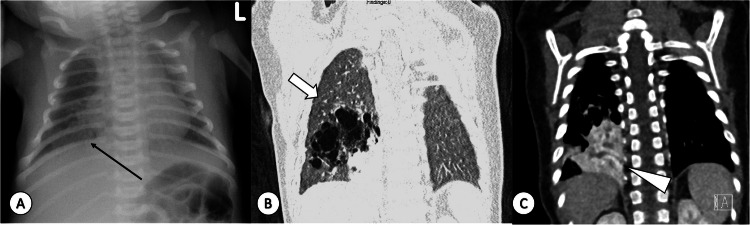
Mixed lesion comprising congenital pulmonary airway malformation (CPAM) type 2 and Intralobar sequestration (ILS) in a 1-week-old girl. A Antero-posterior (AP) chest radiograph revealing a poorly defined lesion in the right lung base (thin arrow in A). B CT, coronal plane, lung window, highlighting multiple cysts smaller than 2 cm associated with the CPAM type 2 component (thick arrow). C CECT, mediastinal window displaying a soft tissue-enhancing lung lesion in the right lower lobe (arrowhead), supplied by the descending aorta and draining through the pulmonary vein (not shown)
